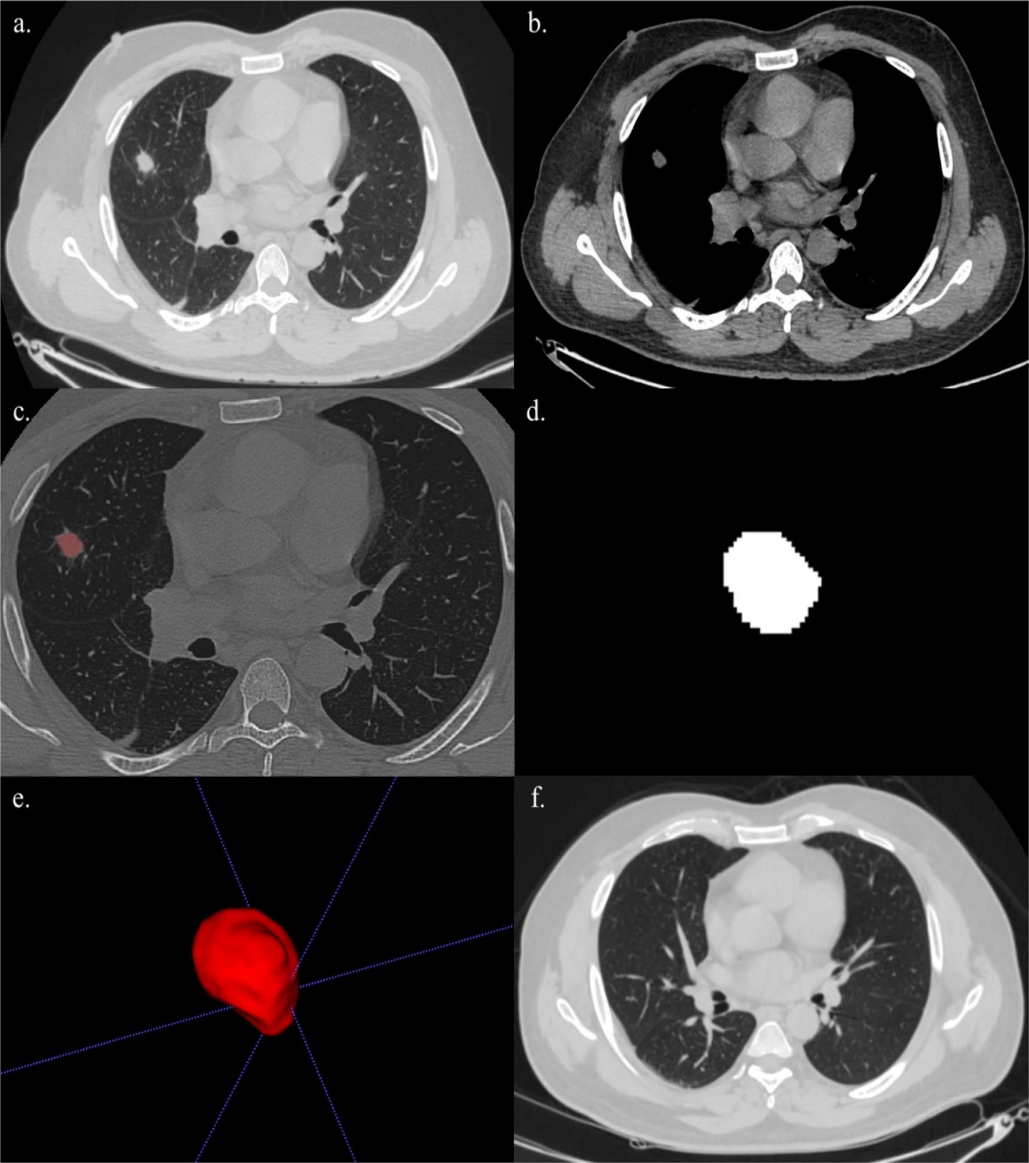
A 53 year-old male with complains of cough and fever. (a, b) Axial section of CT thorax in lung window and soft tissue window showing nodule in the latreral segment of right middle lobe. (c) Creation of ROI for segmentation. (d) 2D segmented nodule. (e) 3D volumetric rendering of the nodule. (f) Patient was put on a course of antibiotic therapy and follow-up imaging done after 6 months revealed complete resolution of the nodule.
